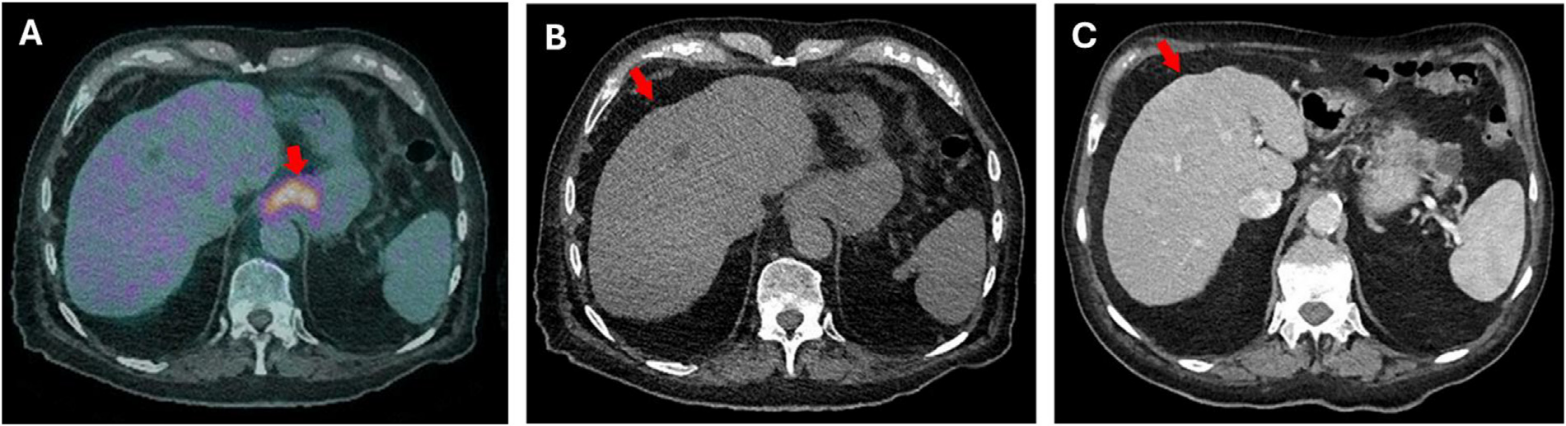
(A) Axial 18F-FDG PET image at the level of the gastroesophageal junction (GOJ) demonstrates intense FDG avidity corresponding to the primary GOJ malignancy as indicated by the red arrow. (B) Axial low-dose noncontrast CT (from PET/CT) demonstrates nodular hepatic contours without discrete focal hepatic lesions indicated by the red arrows and (C) Axial postcontrast CT of the liver shows nodular liver margins indicated by the red arrows, with no evidence of focal parenchymal lesions.
